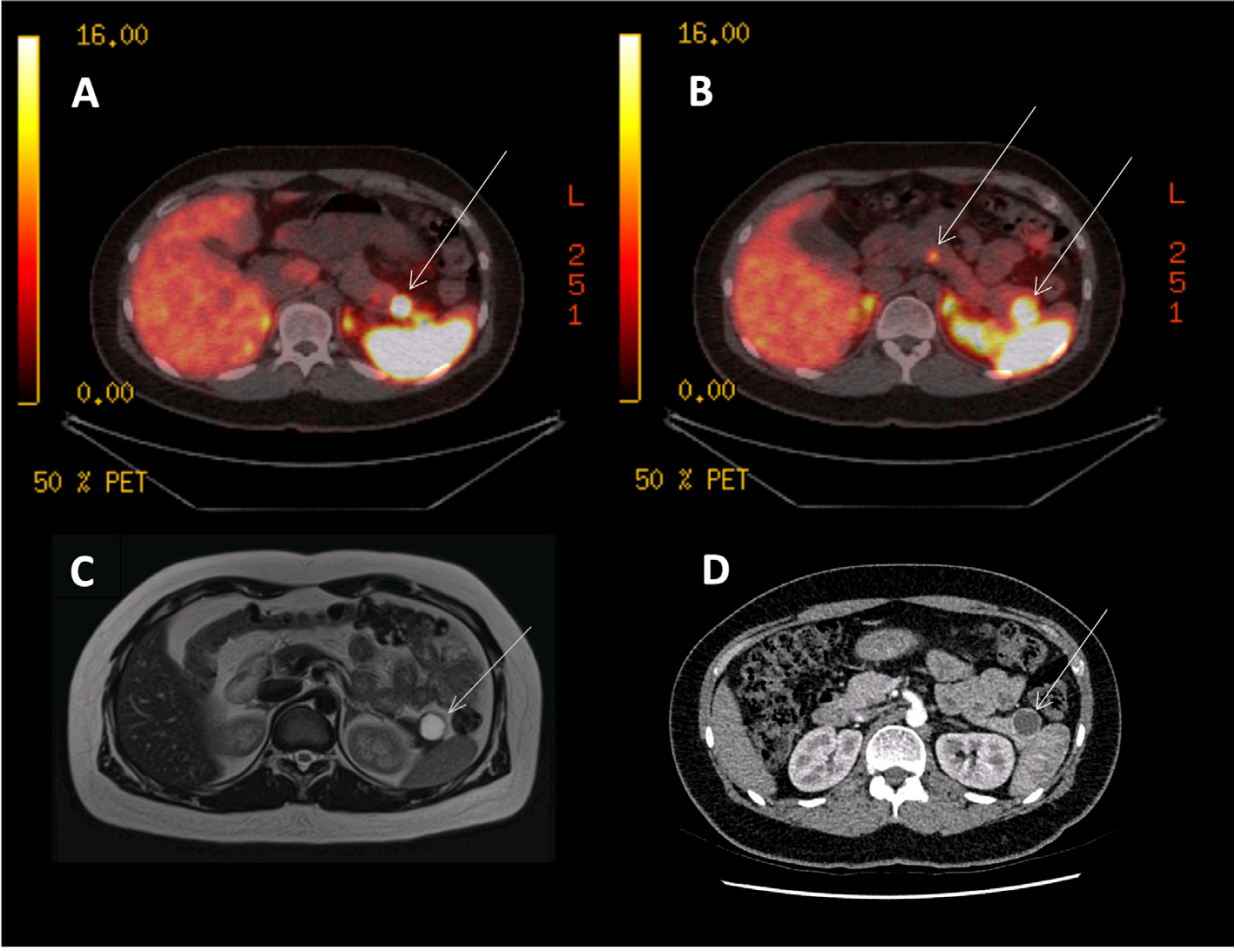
^68^Ga-DOTANOC PET, CT and MRI images of an MEN1 patient with three panNETs (T3N0M0). (A)
